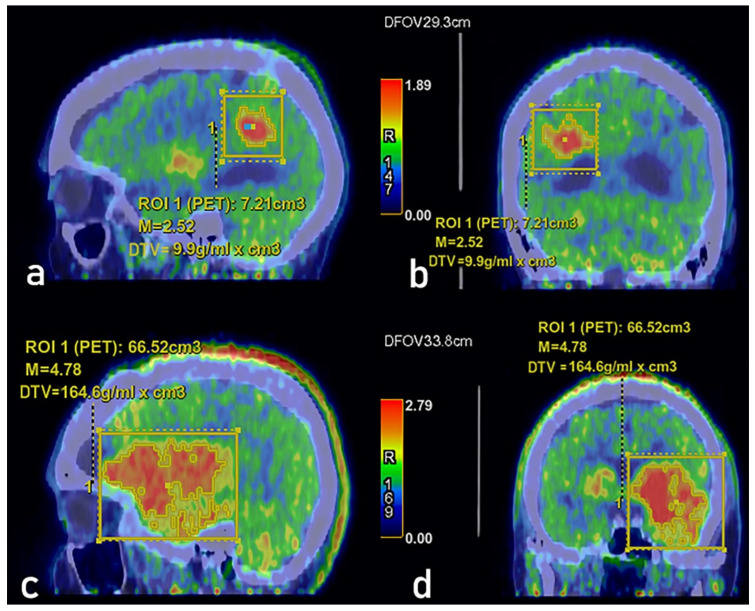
Dopaminergic tumor volume (indicated as DTV in the figure) calculation on a dedicated workstation (version 4.4, Advantage Workstation, GE Healthcare, Chicago, IL, USA) in a patient with Grade II glioma in sagittal (a) and coronal (b) views, and in a patient with Grade IV glioblastoma in sagittal (c) and coronal (d) views. The values of DTV are correlated to SUV max (see the text).
